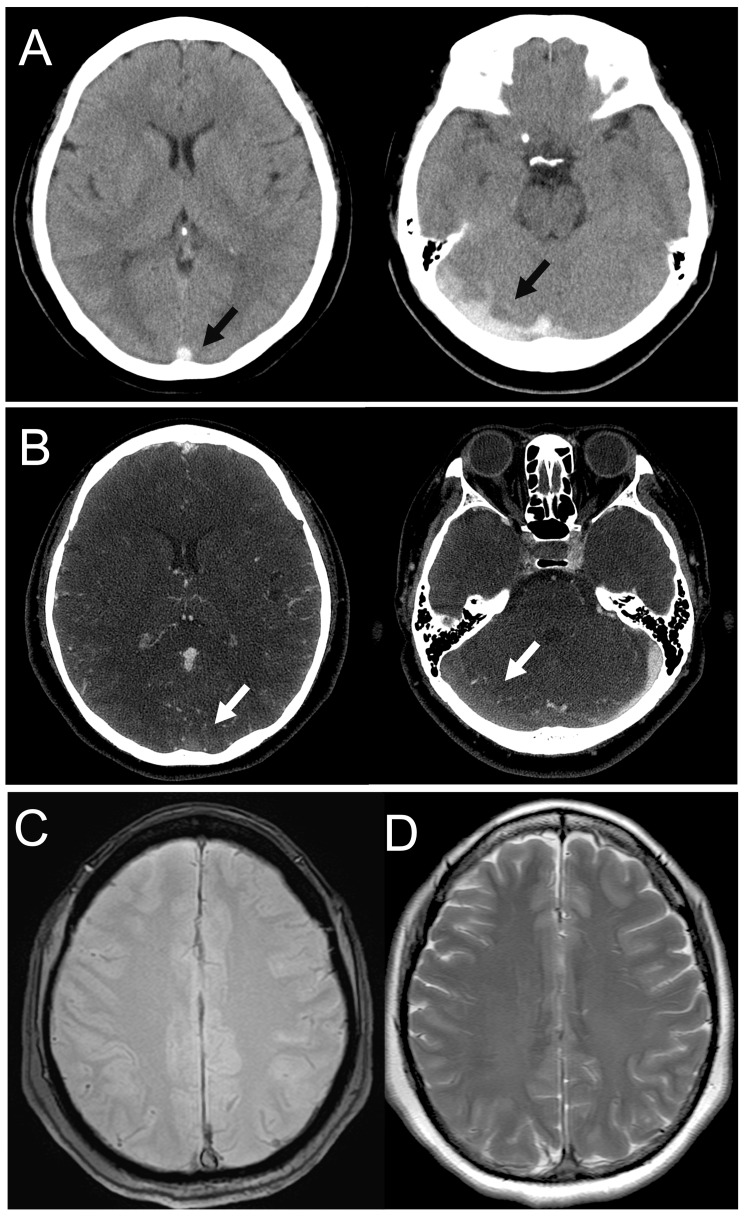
Imaging of CVST on admission Axial CT scans (upper row), CT venography (middle row), and MRI images (bottom row) obtained upon admission are shown. A dense clot sign (black arrows) and a filling defect (white arrows) in the superior sagittal and right transverse sinuses were suggestive of CVST (A, B). MR imaging at the supratentorial level shows no cerebral edema and intracranial hemorrhage (C: T2*, D: T2-weighted image). CVST - cerebral venous sinus thrombosis
